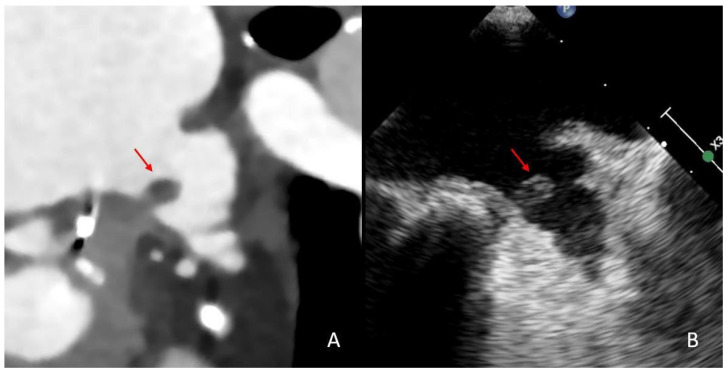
Comparison of thrombus imaging in the LAA on cardiac CT and transesophageal echocardiography in the same patient. This patient presented with an acute stroke with occlusion of the basilar artery. Upon cCT imaging, a thrombus can be depicted proximally in the LAA (red arrow, image (A)). The thrombus was still detectable on TEE three days after the stroke event (red arrow, image (B)).
